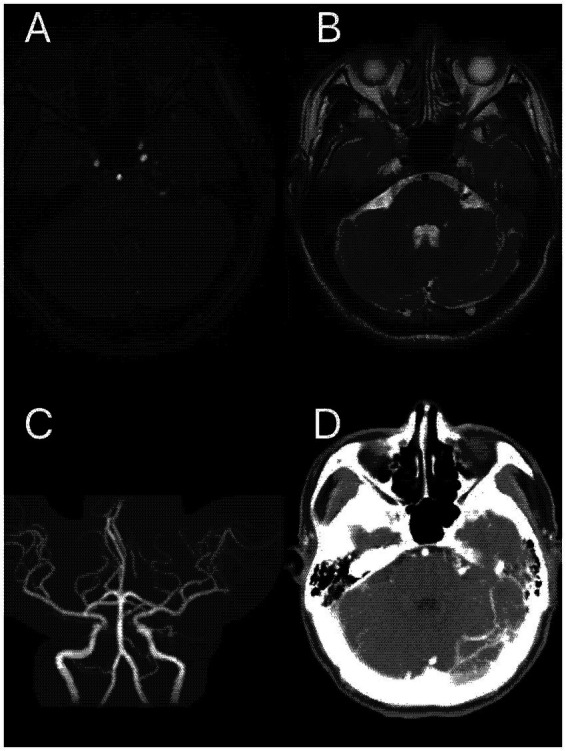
A malformed vascular mass in the left pontine cerebellar horn region seen on MRI scanning (A,B); Panel (C) is magnetic resonance angiography (MRA); and Panel (D) is unreconstructed CTA showing a clear vascular malformation.
